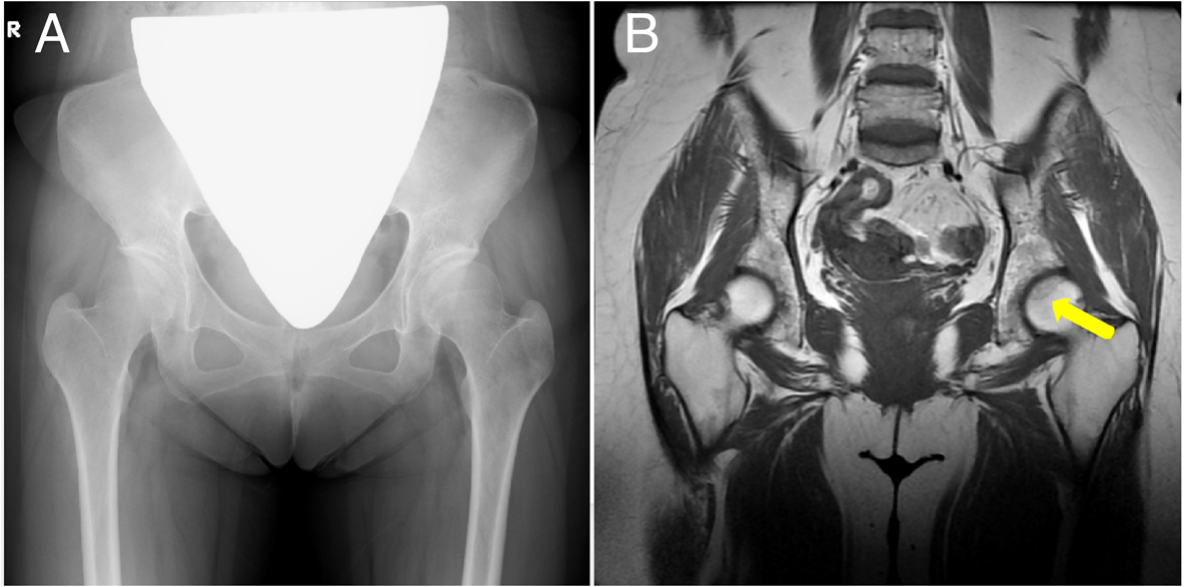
Images obtained at the same month of the onset of hip pain. (a) Anteroposterior radiograph of the hip at the first visit. (b) Coronal T1-weighted magnetic resonance image of the hip in the same month. Low-intensity band pattern in the left femoral head (arrow)
